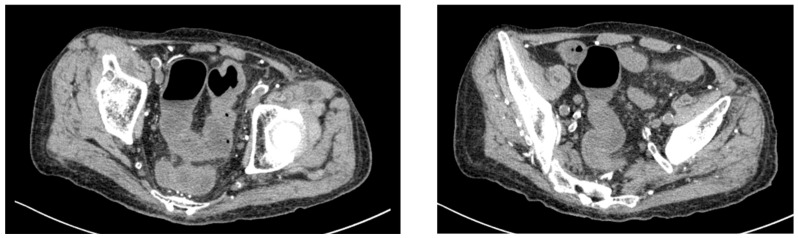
Abdominal computed tomography findings at emergency department presentation. The CT scan demonstrates thickening of the colonic wall.
